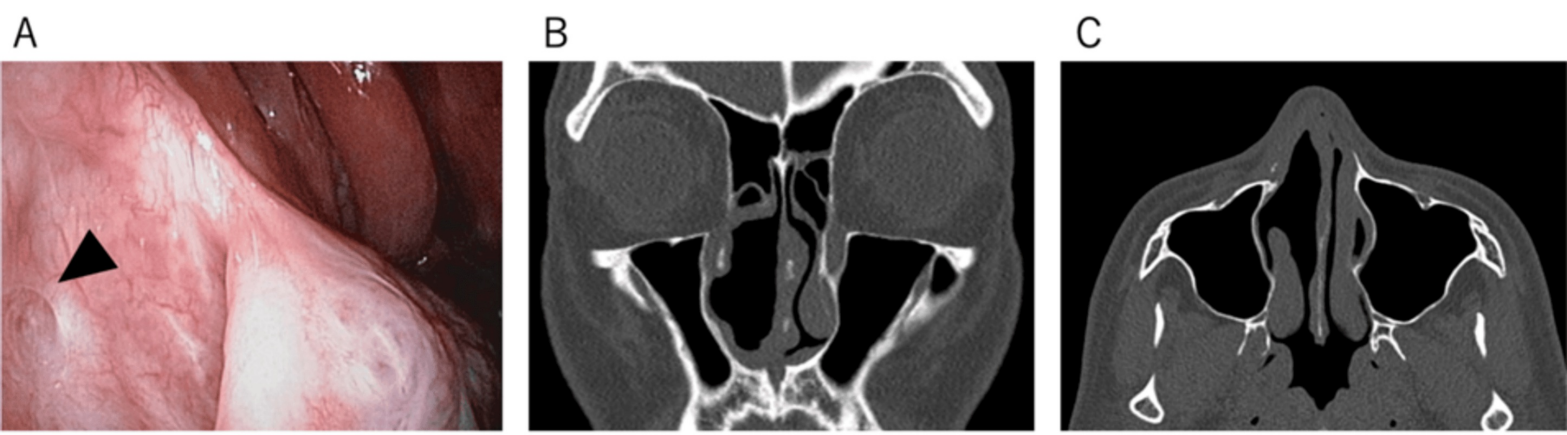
Findings at the 12-Month Postoperative Follow-Up (A) No evidence of tumor recurrence. The black arrowhead indicates the opening of the nasolacrimal duct. (B-C) CT images show no radiological findings suggestive of recurrence. CT, computed tomography
